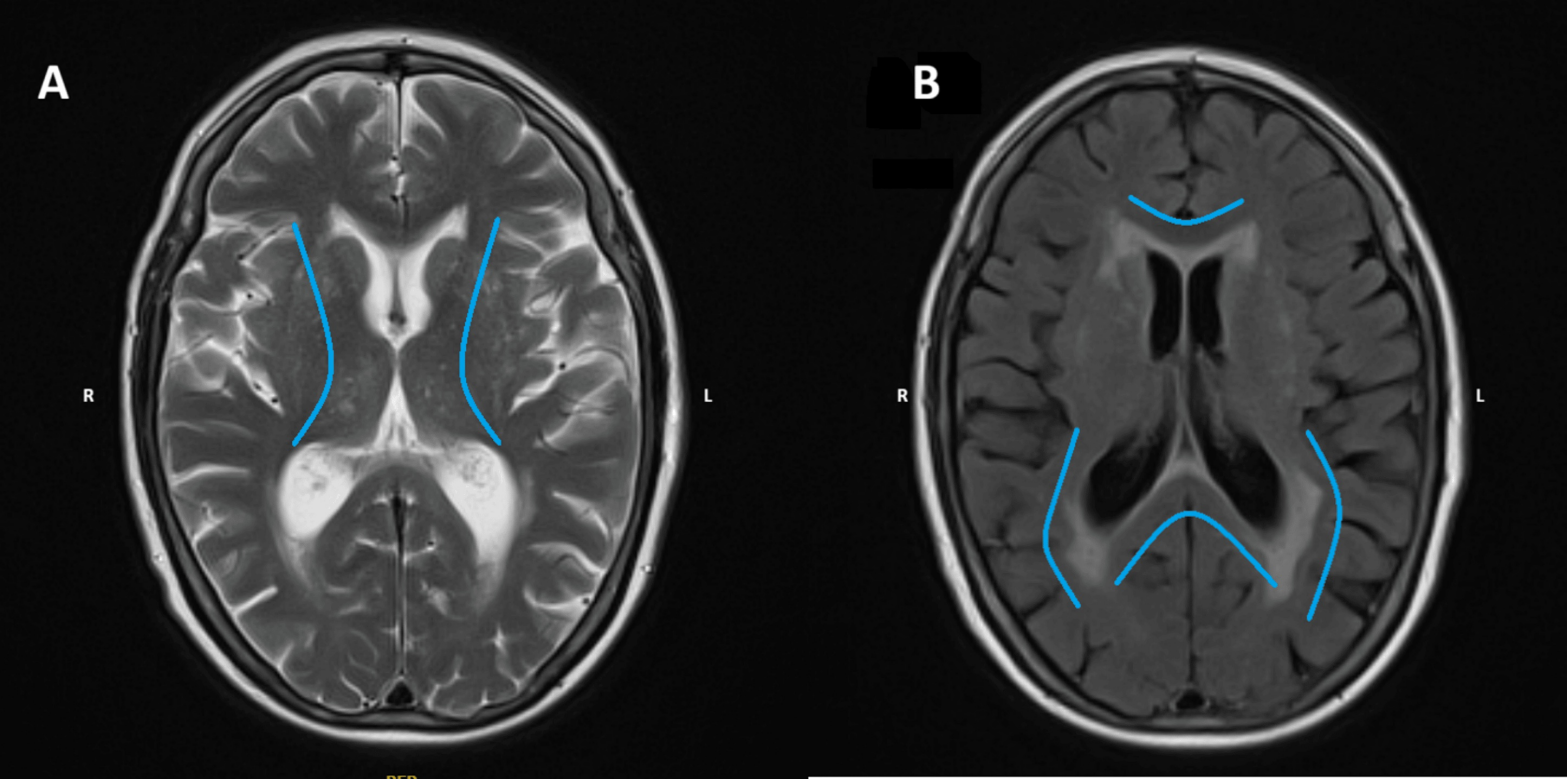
MRI head showing the chronic white matter changes Axial MRI brain images demonstrating chronic changes. (A) T2-weighted image. (B) Corresponding FLAIR sequence showing chronic white matter changes (in blue) FLAIR: fluid-attenuated inversion recovery The images have been marked with right (R) and left (L) for orientation.
